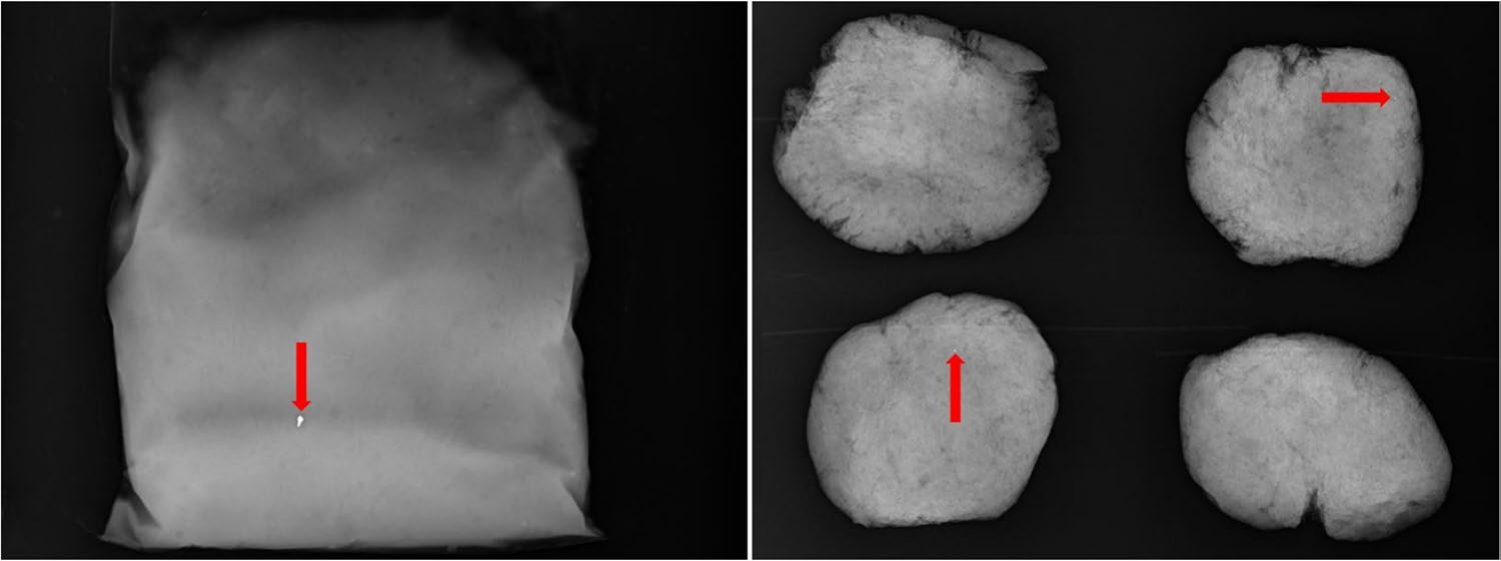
Radiographs of Australian game meat contaminated with metallic fragments (red arrows) assumed to be lead. The radiograph on the left shows a commercially available packet of wallaby mince containing a metallic fragment 3.7 mm long. The radiograph on the right shows four burgers made from deer meat (venison) by a recreational hunter, two of which contain metallic fragments 0.6–0.7 mm long
